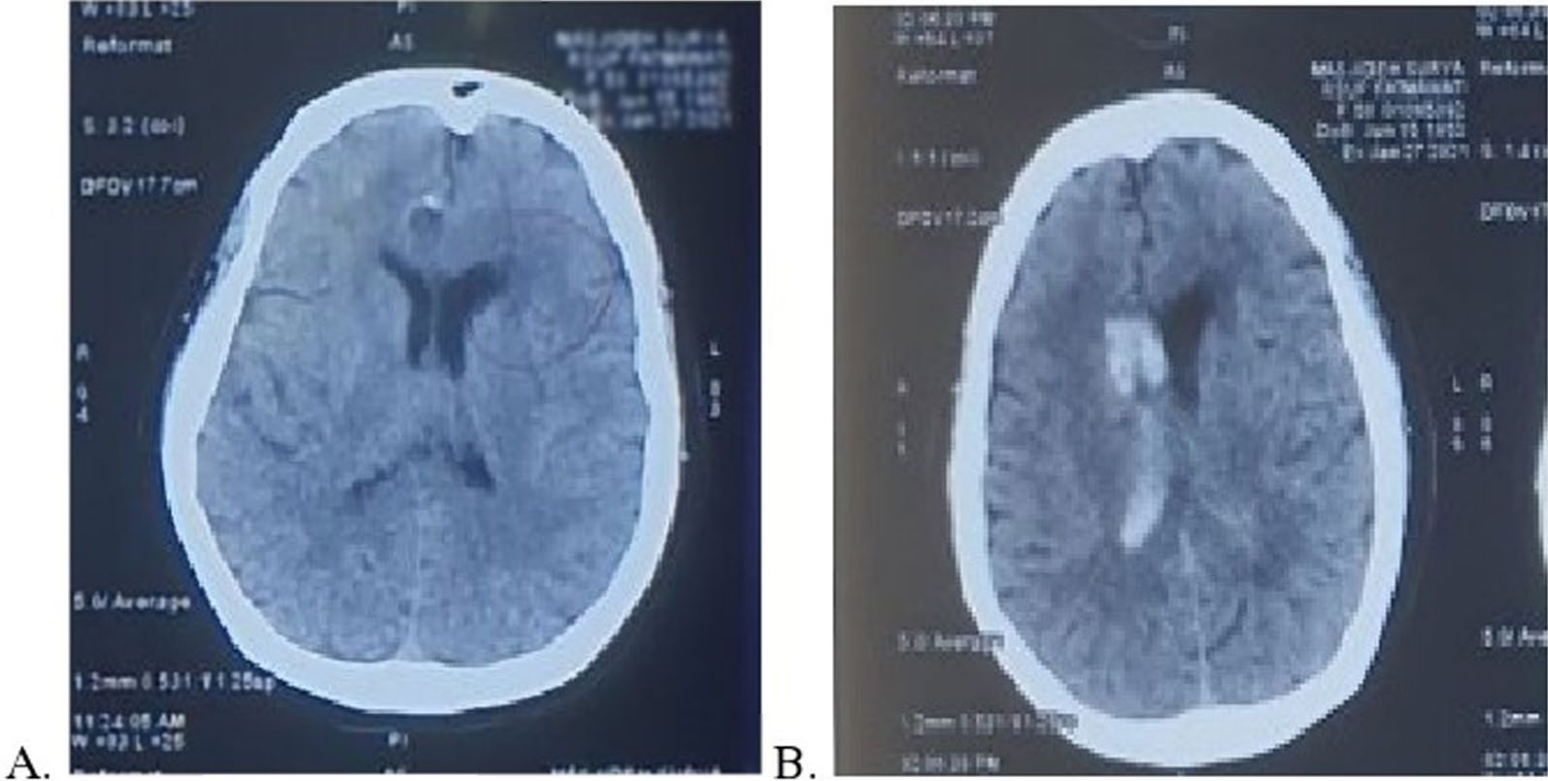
Head CT Scan A Before thrombolysis showed infarct in capsula eksterna sinistra cornu anterior, B After thrombolysis showed bleeding in lateral ventricle bilateral especially in the right and third ventricle
